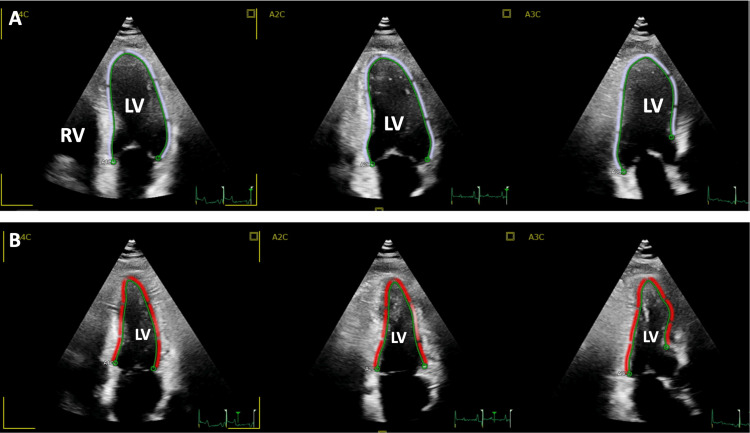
Apical echocardiographic views showing normal left ventricular function Echocardiographic apical four-chamber views at end-diastole (A) and end-systole (B) demonstrate normal wall motion of the LV without evidence of contractility or valvular abnormalities. LV: left ventricle; RV: right ventricle
